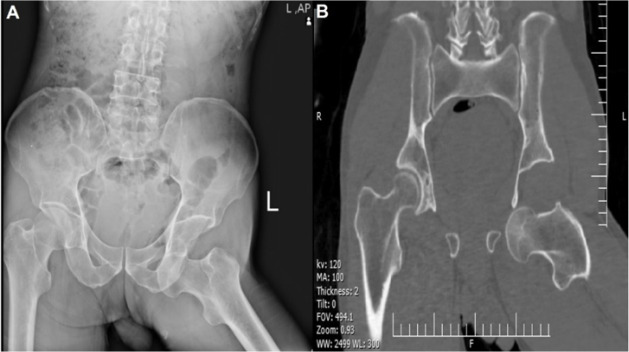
(A) Primary pelvic x-ray radiograph, (B) Pre-reduction computed tomography (CT) scan
